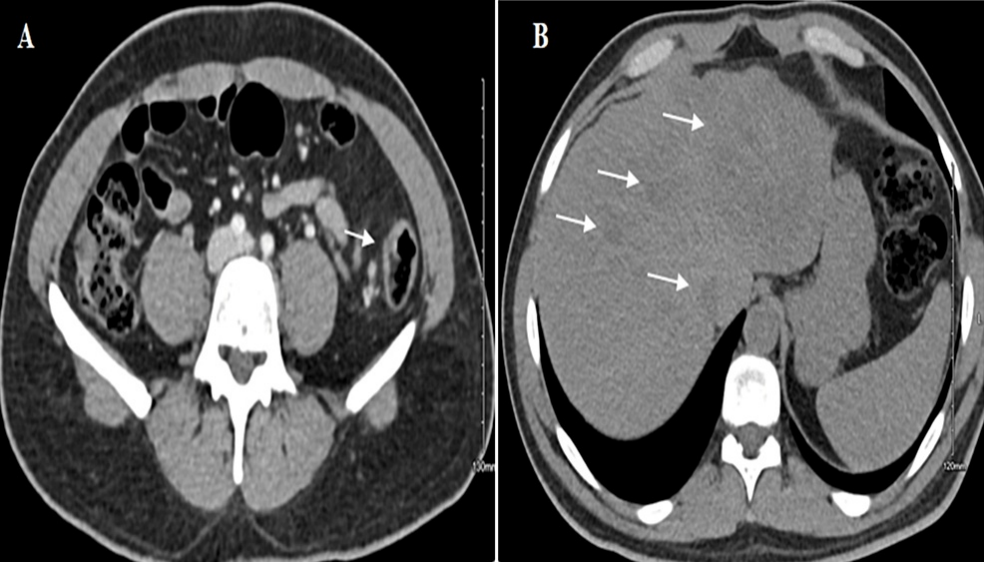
Enhanced axial CT of the abdomen and pelvis. Contrast-enhanced axial images of the abdomen and pelvis show (A) a nonobstructive colonic sigmoid mass with locoregional lymphadenopathy. In addition, (B) multiple innumerable hepatic lesions highly suspicious for metastasis are noted.
